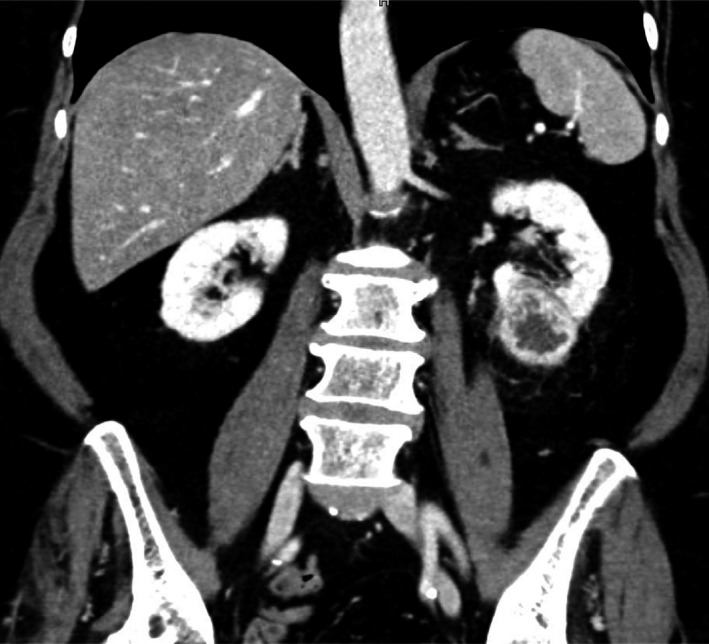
CT scan, preoperative. A 4.2 × 3.6 × 4.3 cm large mass in the lower pole of the left kidney
